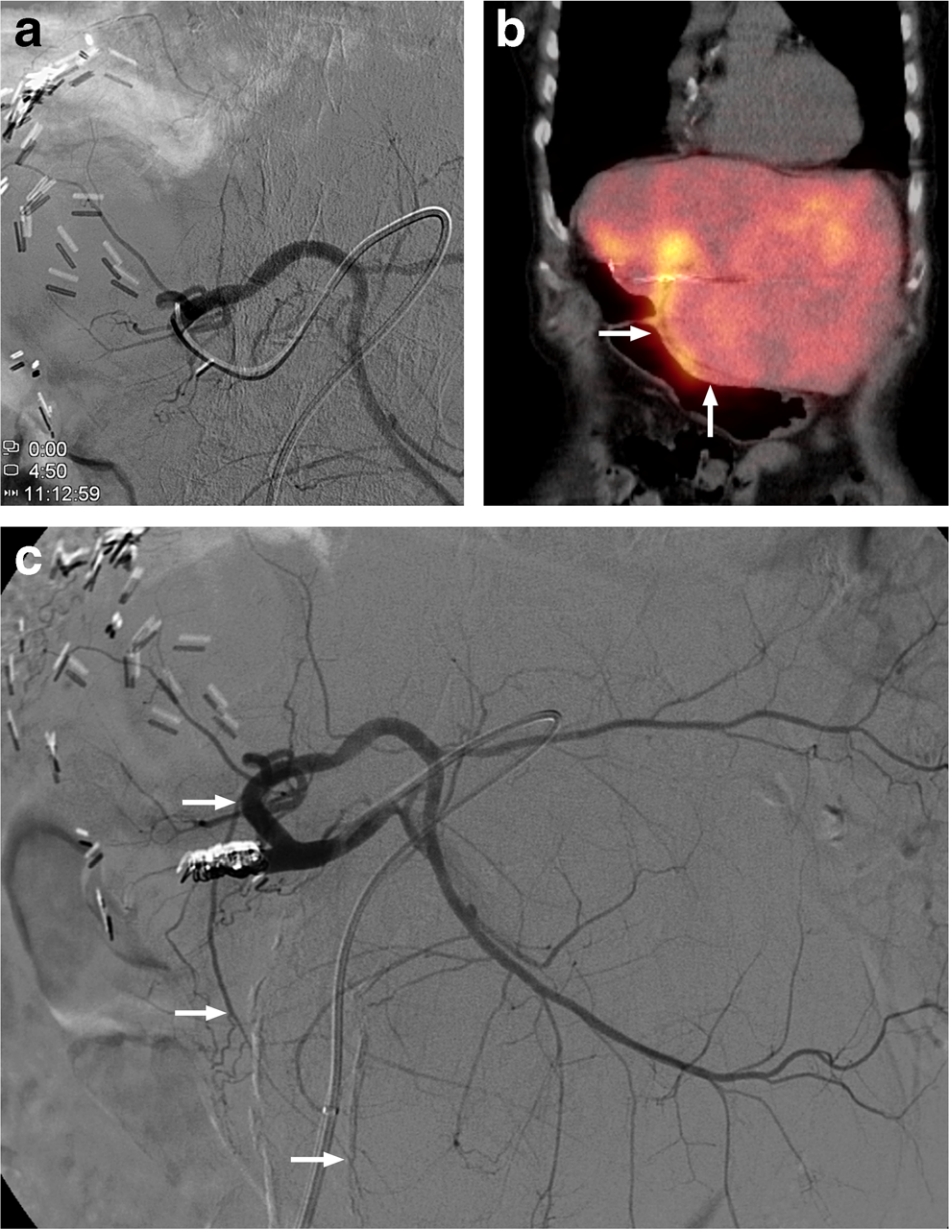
An 80-year-old female with colorectal cancer liver metastases was initially treated with a resection of her sigmoid carcinoma and simultaneous right hemihepatectomy. (a) Digital subtraction angiography (DSA) with injection position during ^166^Ho scout dose pre-treatment angiography. (b) SPECT/CT after administration of ^166^Ho scout dose with large extrahepatic deposition in lesser curvature of the stomach (arrows). (c) DSA showing the right gastric artery arising from the right hepatic artery as culprit vessel (arrows)
